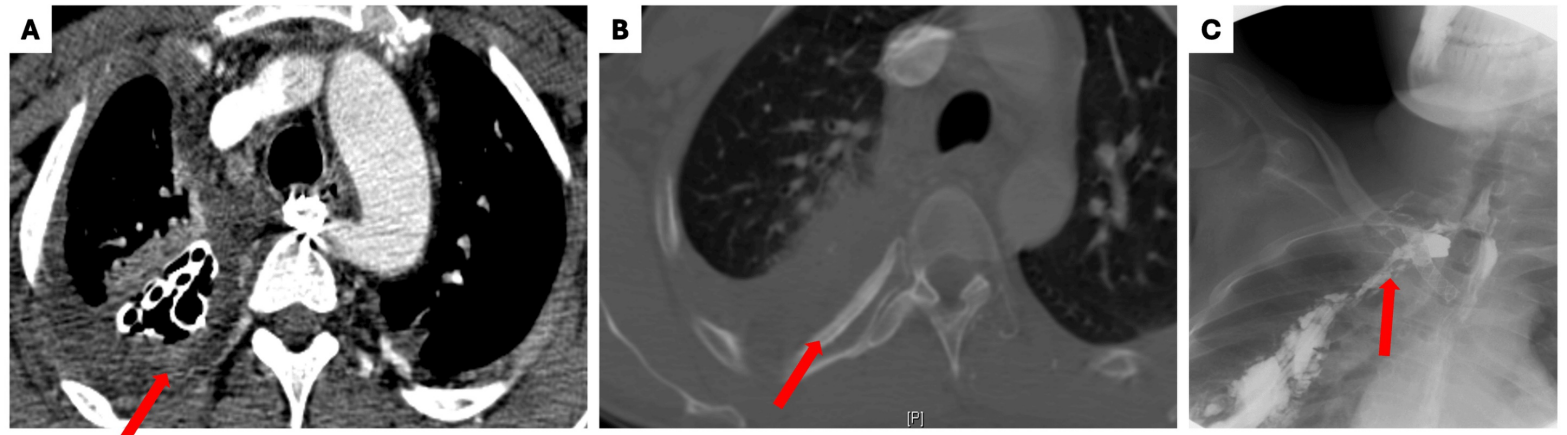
Imaging studies after eight months that indicated stent infection, periosteal reaction, and esophageal leak (A) Axial computed tomography angiogram (CTA) with intravenous contrast in soft tissue window demonstrating aberrant right subclavian artery (ARSA) stent. There is fluid collection surrounding the stent (red arrow) and also in the right pleural space, concerning for stent infection; (B) Bone window of the CTA demonstrating periosteal reaction (red arrow), indicating bone involvement leading to osteomyelitis; (C) Upper gastrointestinal fluoroscopic study demonstrating esophageal leak along the ARSA stent into the right pleural space (red arrow).
